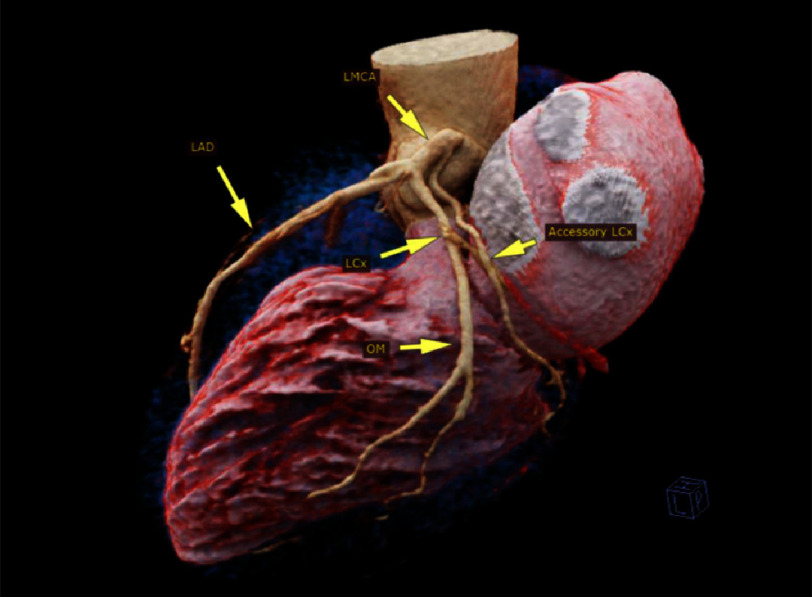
Volume-rendered computed tomography of the heart showing the origin and course of main and acessory circumflex artery. LMCA: left main coronary artery, LAD: left anterior descending, LCx: left circumflex, OM: obtuse marginal.
